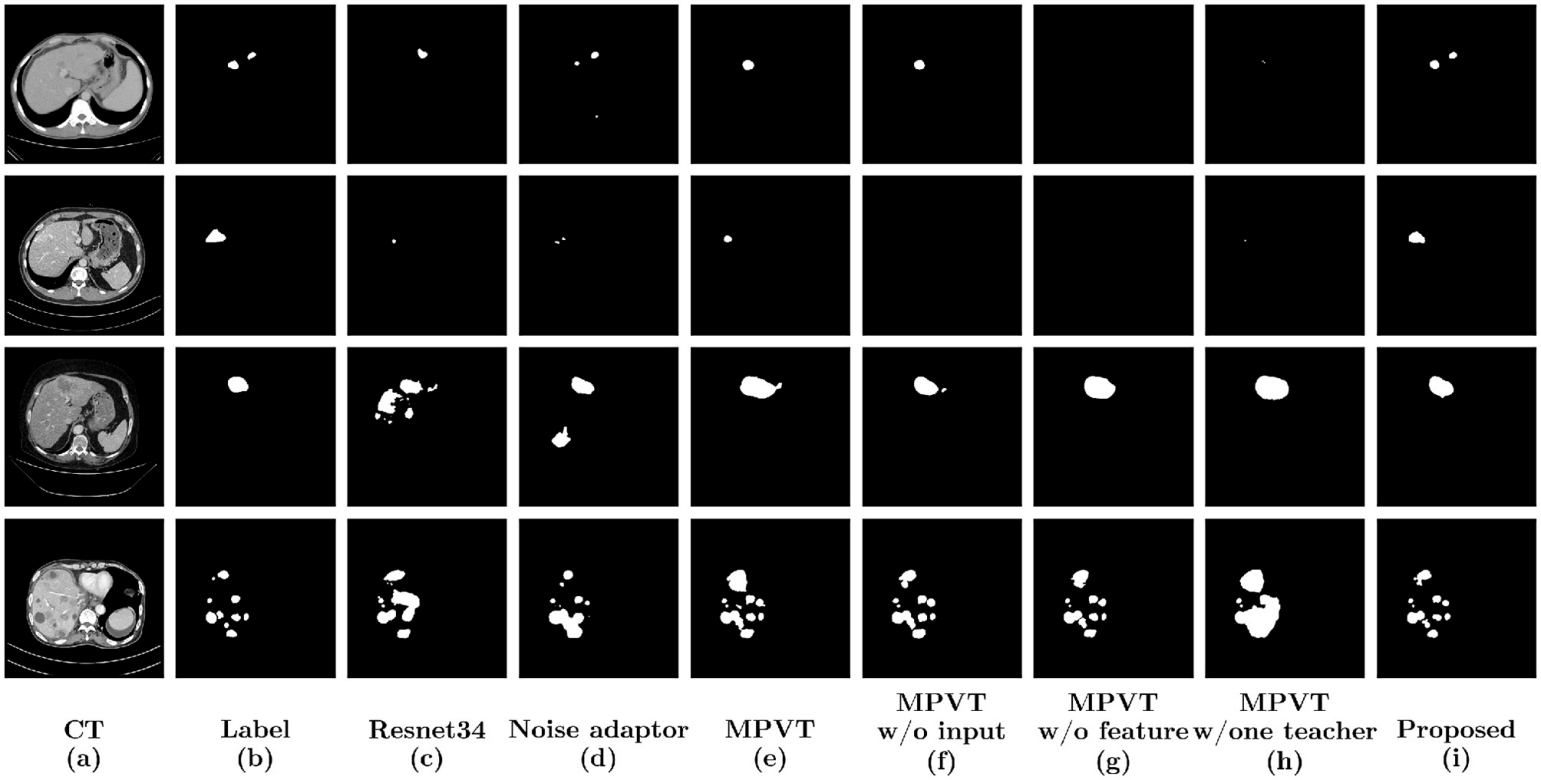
Display of results of ablation analysis. Four samples were selected to display the results of the six methods. (a) Original CT images; (b) Labels; (c) Results of U-Net with a backbone structure Resnet34; (d) Results of training with noise adaptor; (e) Results of MPVT; (f) Results of MPVT without input perturbations; (g) Results of MPVT without feature perturbations; (h) Results of MPVT with only one teacher model; (i) Results of our method.
